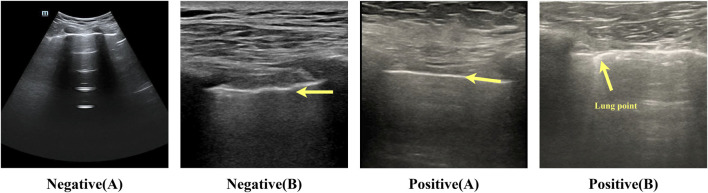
Example of negative-positive data. Negative (A): lung sliding feature, white pleural lines that slide regularly with respiration. The bat sign is a normal ultrasound manifestation in the lungs. Negative (B): lung pulse feature, bright white pleural lines indicated by the arrows in the figure, appear as wavy lines in response to the heartbeat. Positive (A): no lung sliding lung booting feature; bright white pleural lines indicated by the arrows in the figure are straight and non-displaced or in a relatively static state. Positive (B): lung pointing feature; pleural lines appear as discontinuous breakpoints, half of which are sliding and generally not sliding.
